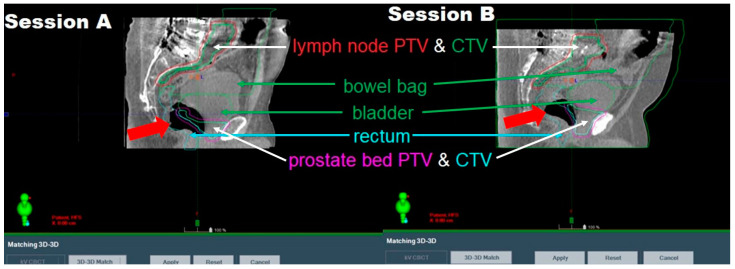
Two sample aborted sessions (Session A and Session B) due to large intrafractional gas changes for two different patients receiving prostate bed oART. Shown are the post-planning CBCT acquired immediately prior to treatment delivery superimposed with the on-couch contours delineated based on the pre-planning CBCT at the start of the oART session. The color-coded legends point to the oART contours and not the shown anatomy. In both cases, big gas bubbles (indicated by the big red arrows) substantially deformed the prostate bed targets and OARs like rectum and bladder, leading to session abortion.
